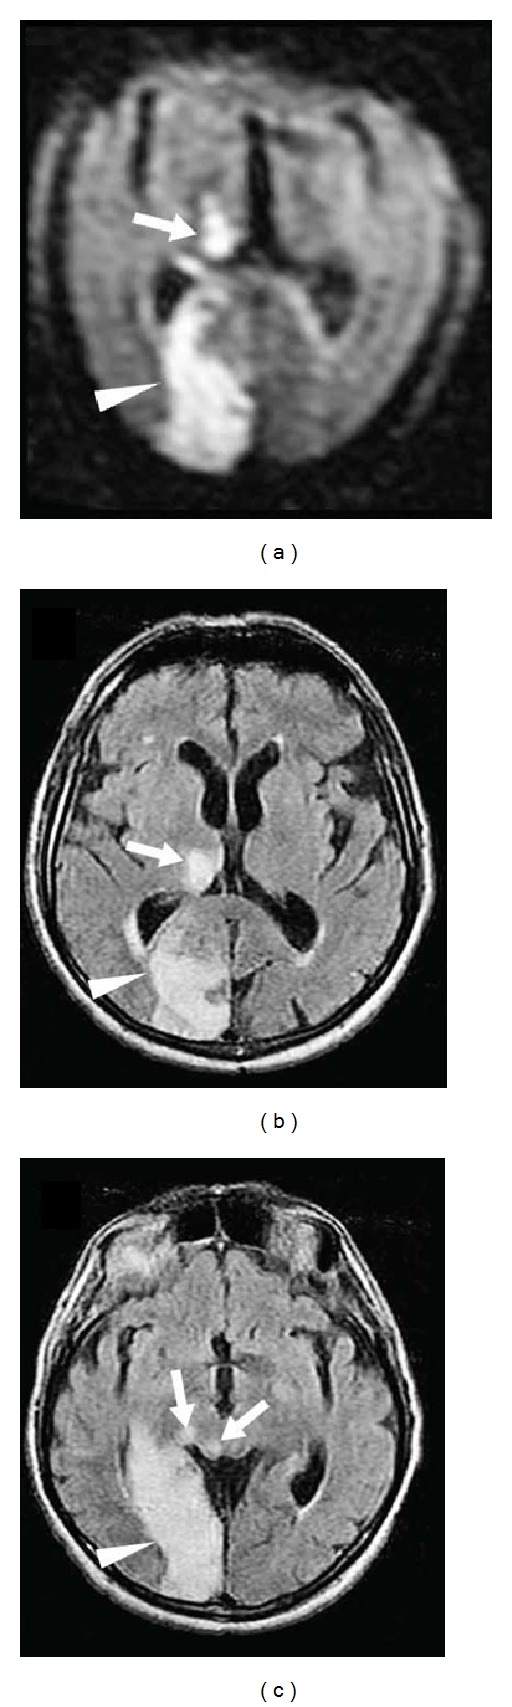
DWI (a) and FLAIR (b and c) imaging showing infarctions involving the territories of the right-sided posterior choroidal artery (a and b, arrows), the posterior cerebral artery (a, b, and c, arrowheads), the collicular artery, and the posteromedial choroidal artery (c, arrows).
